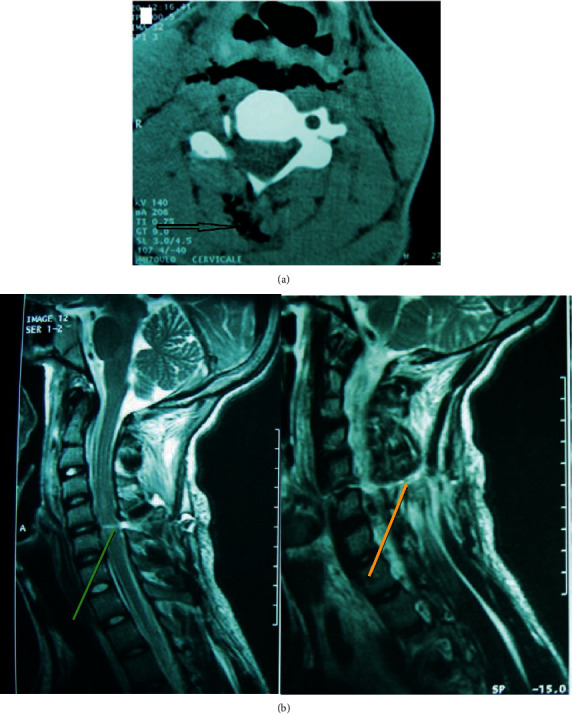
(a) CT scan, axial section at C4C5, showing hypodensity in the posterior left-sided soft tissue representing the knife track with its passage filled with air (black arrow). (b) MRI sagittal T2-weighted hyperintense signal of the spinal cord at C4C5 level sign of medullary contusion (green arrow), with the continuous posterior soft tissue hyperintense representing the knife track (yellow arrow).
